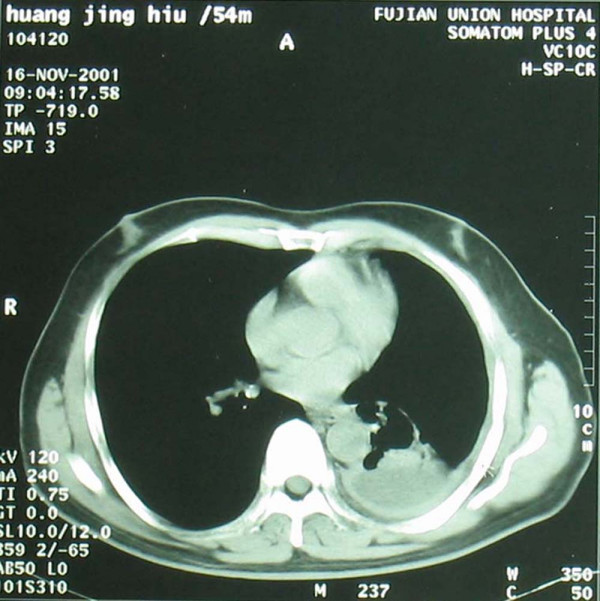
Chest CT after treatment (16-Nov-2001) show that clear shrinkage of conglomeration of 3.0 × 2.5 cm at the left lower hilus pulmonis, small amount of accumulation of fluid in the left thoracic cavity.
